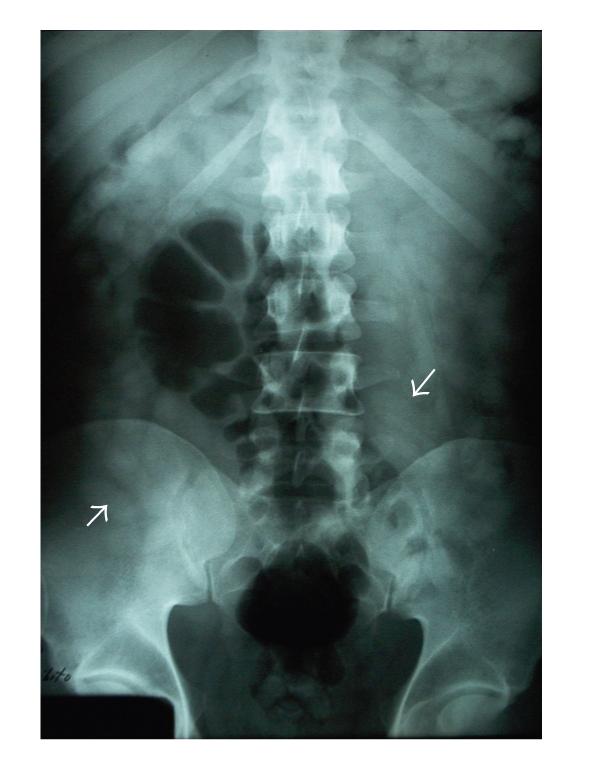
Multiple foreign bodies in the bowel in plain abdominal radiograph.
